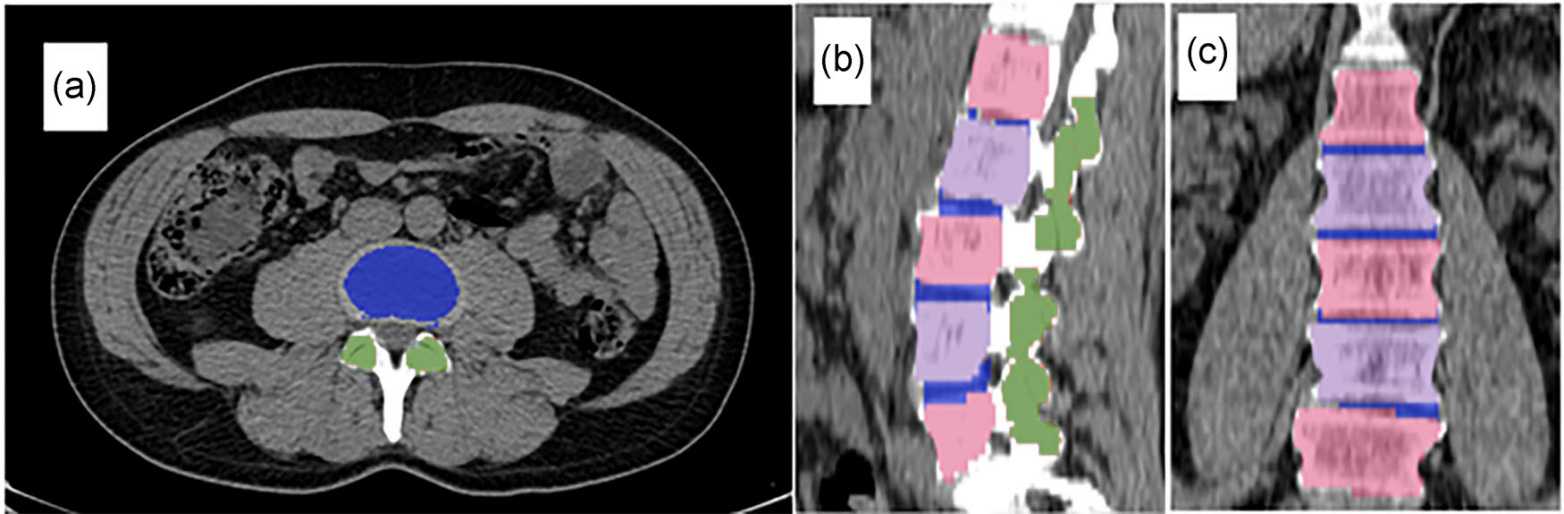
A sample of the semiautomatic segmentation shown in the computed tomography images in transaxial (a), sagittal (b) and coronal (c) planes. Vertebral bodies are shown with intermittent light blue and light red colours, facets with dark green colour and intervertebral discs with dark blue.
